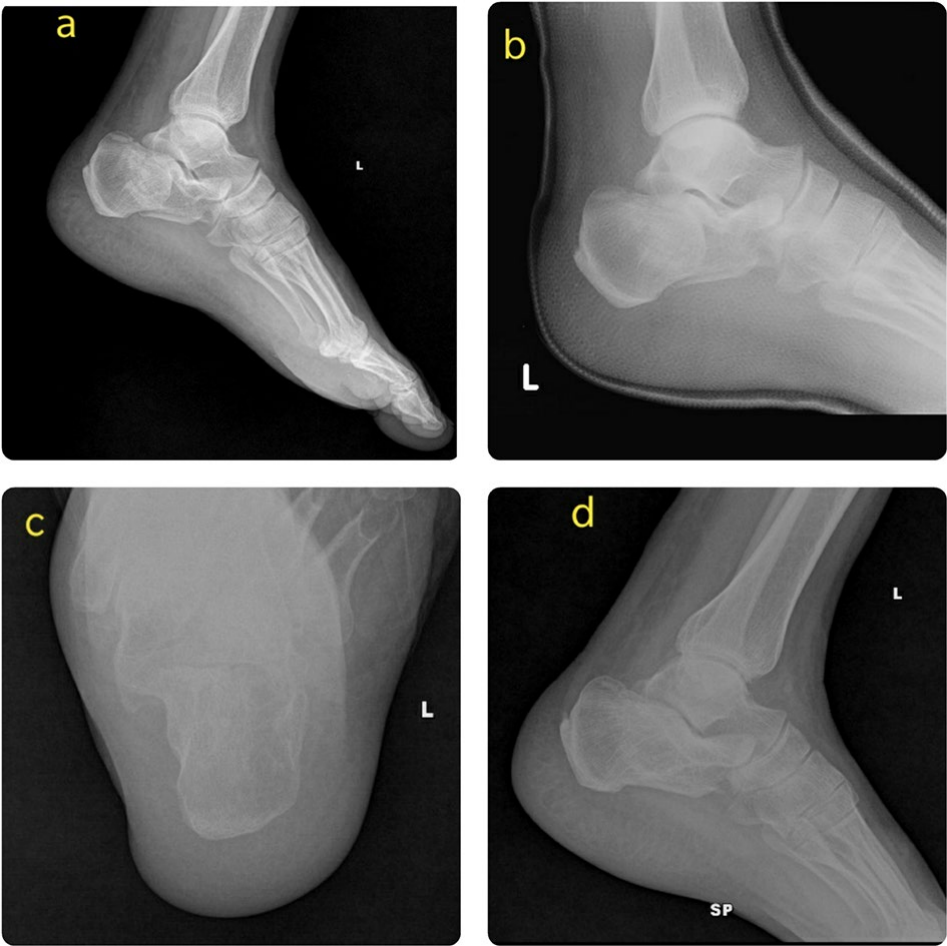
Calcaneal fracture treated by non-operative method. (a) Post injury radiograph, (b) radiograph after casting, (c,d) radiographs at one year follow up.
